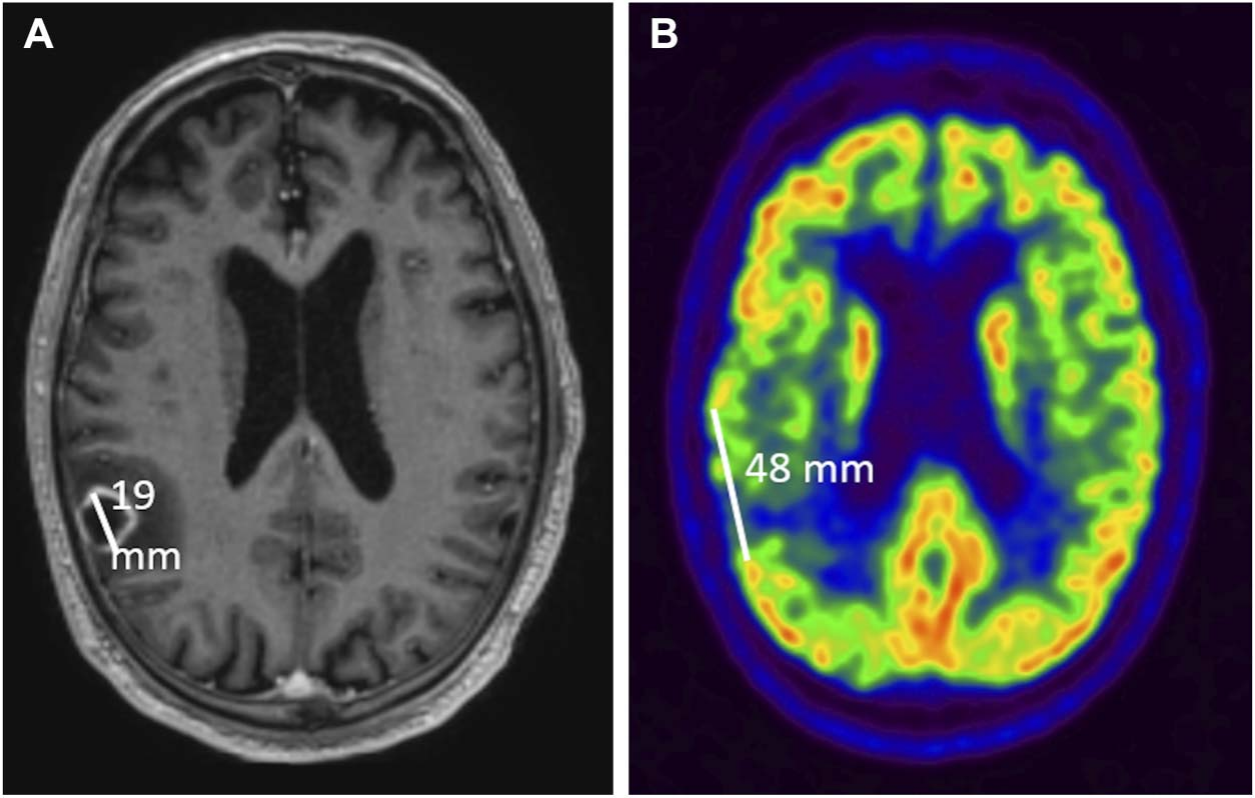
Measurement of abscess dimension and extent of neocortical hypometabolism. A, T1-weighted MRI shows the maximal diameter of the abscess in right parietal lobe in the horizontal plane. B, [^18^F]-fluoro-2-deoxy-D-glucose positron emission tomography/computed tomography in the same plane as image a, obtained 20 months later, shows the extent of neocortical hypometabolism in the area where the abscess was located. Note that the hypometabolic area exceeds the dimensions of the abscess.
